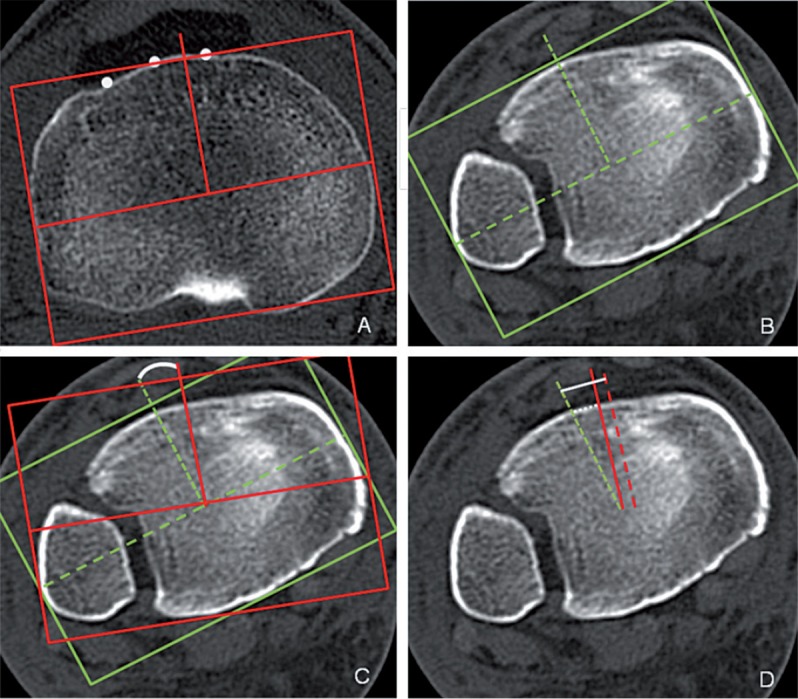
A. Axial scan showing proximal AP axis 1 passing through one-third of the tibial tuberosity (TT). B. Distal AP axis, i.e. the projection of the center of the ankle joint. C. Axial scan showing the mismatch in rotation alignment between proximal AP axis 1 (continuous red line) and the distal AP axis (dotted green line), the latter being externally rotated with respect to the former. D. Axial CT scan showing the extent to which, on average, the reference point of the ankle joint should be translated medially to compensate for tibial torsion (white dotted line) and for tibial torsion plus the difference in malleolar width (white continuous line).
